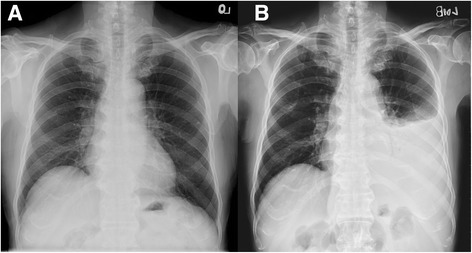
Chest X-rays showed normal heart size, sharp cardiopleural angle, bare stent of coronary artery and no significant of aortic lesion (Panel a). Chest X-rays revealed left pleural effusion and widening of upper descending aorta (Panel b)
